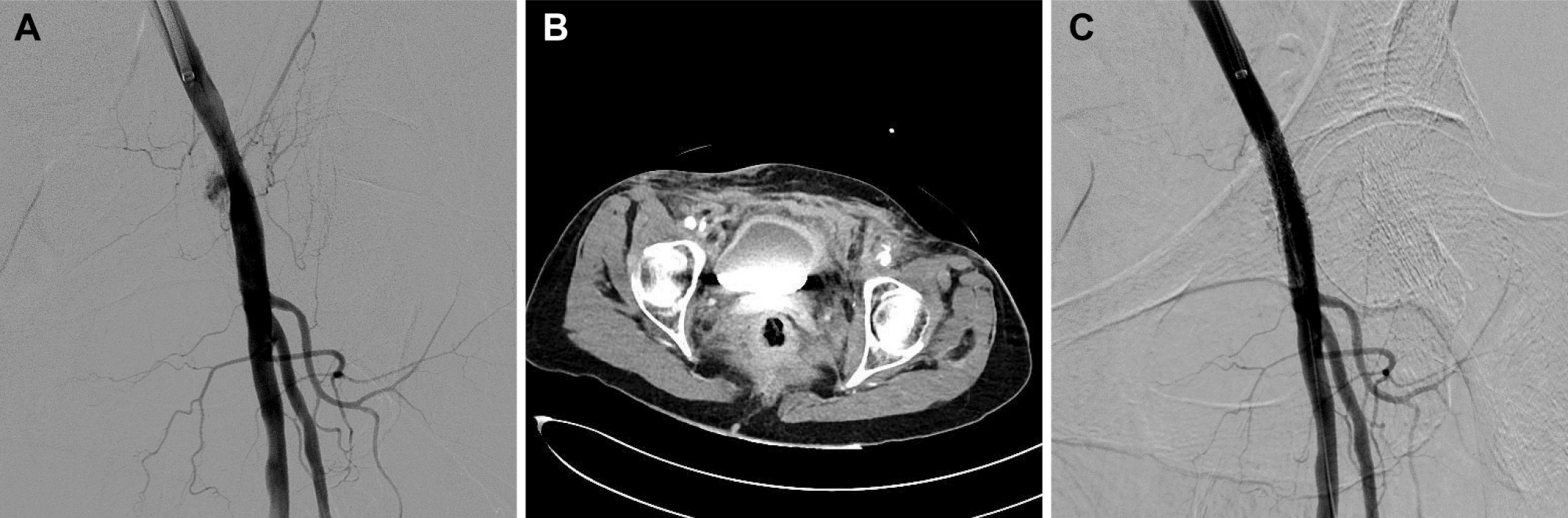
A 66-year-old female experienced a high retrograde puncture above the inguinal ligament, 2.5 cm cranial to the femoral head. Despite manual compression, a rapidly increasing groin haematoma developed. (a) Angiography demonstrated active extravasation from the iliac external artery, immediately above the level of the inguinal ligament. (b) Contrast-enhanced computed tomography scans demonstrated active extravasation from the external iliac artery and an accompanying haematoma. (c) Successful sealing of the perforation was achieved by deployment of an 8 x 26 mm covered stent (LIFESTREAM; C. R. Bard, USA) via a contralateral crossover approach.
